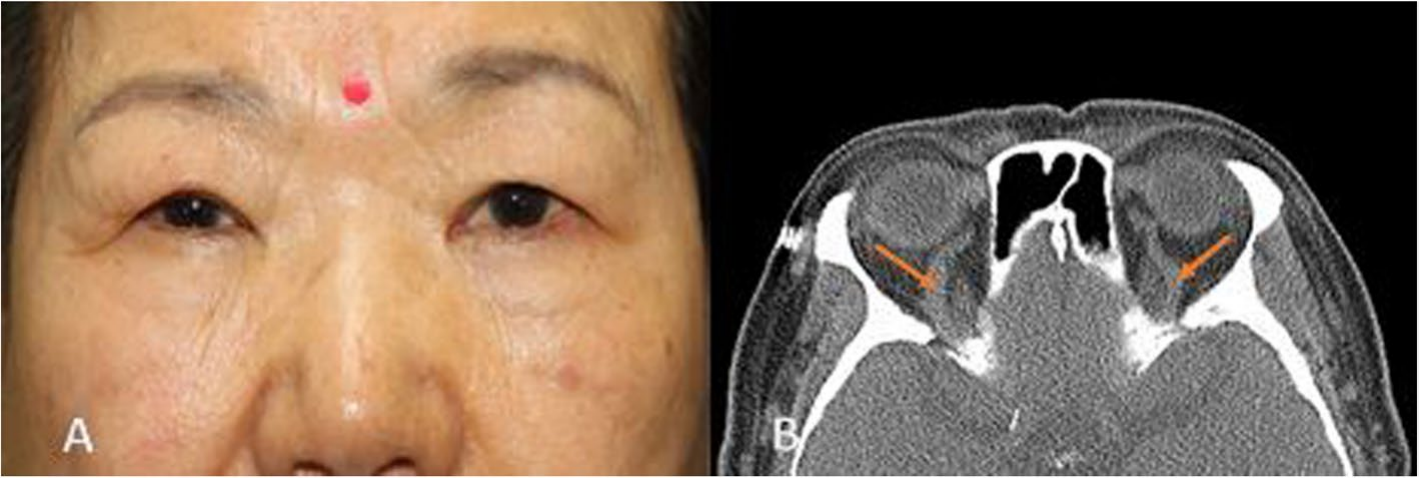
Photographs and CT image of the case 2 patient. A Asymmetric eyelid swellings (right > left) were noted. B Orbital CT (transverse view) revealed bilateral SOV enlargement (Arrows)
